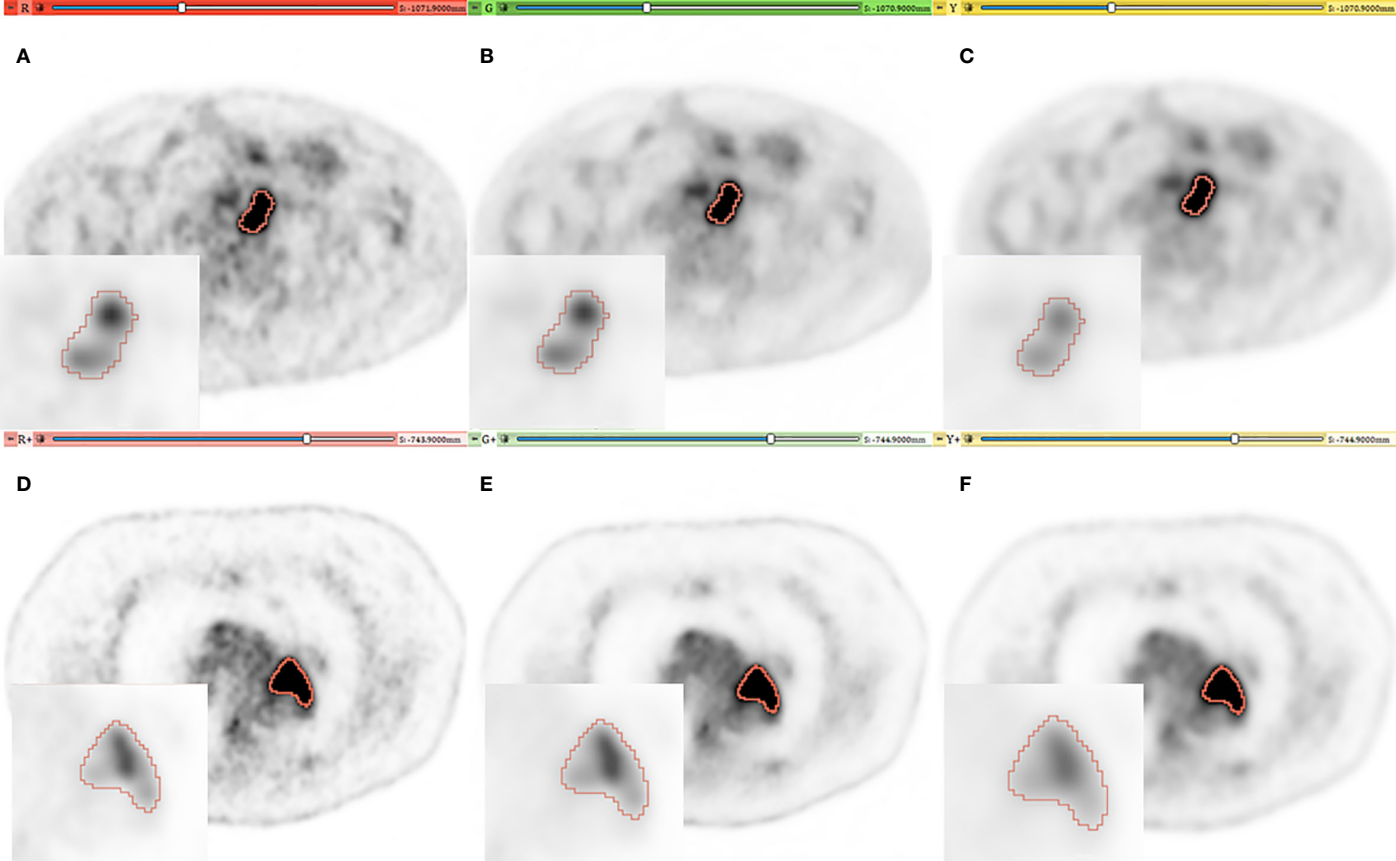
Representative PET imaging of two lesions in different patients with a SUV windowing of (0–5). (A, D) [red], (B, E) [green], (C, F) [yellow] for original, AI and EARL images respectively. A Zoom is added on each image with SUV windowing between (0–25) and (0–30) for the first and second patient.
